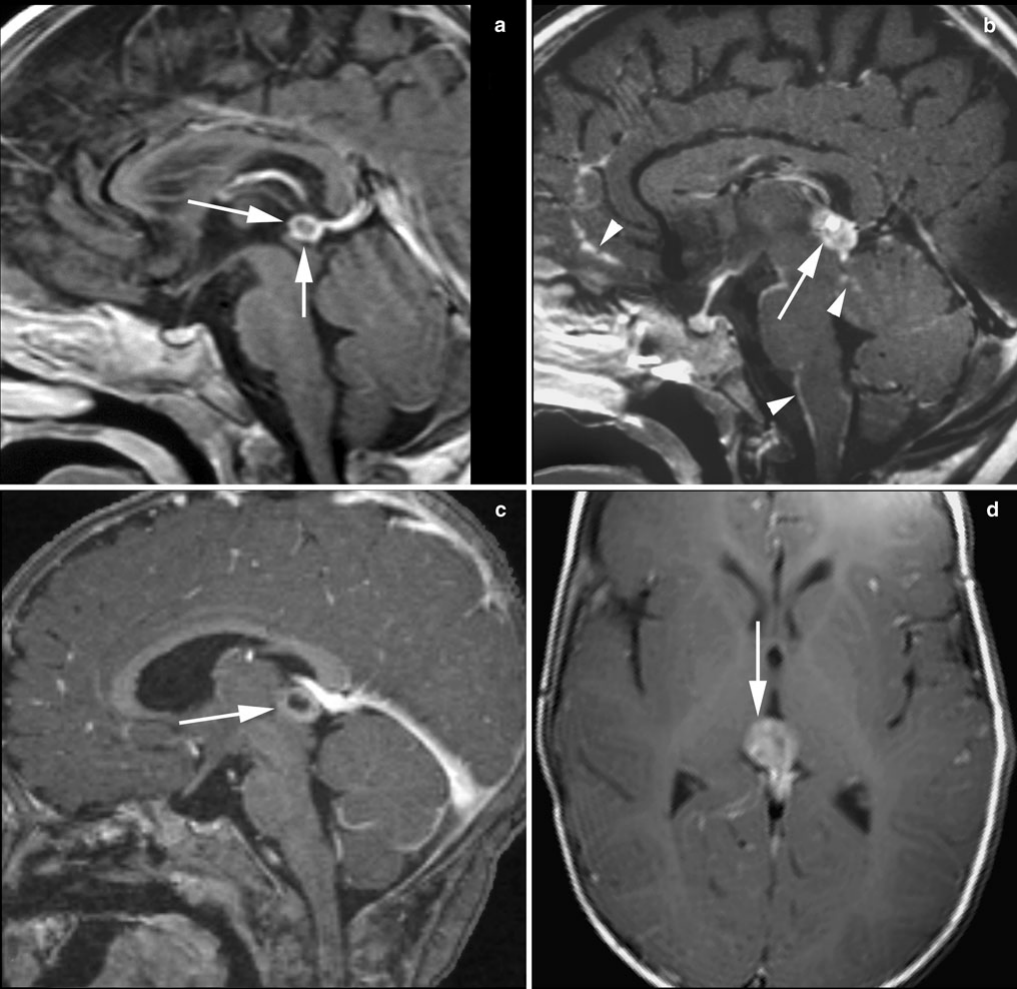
Pineoblastoma presenting as suspicious cyst. Contrast-enhanced sagittal (a–c) and axial (d) T1-weighted images of the brain in patient 6 (a, b) and patient 16 (c, d). The pineal gland in a shows an irregular cyst wall with tiny nodules, which progressed into a solid tumor with diffuse (nodular) leptomeningeal metastases 14 months later after treatment refusal (b). The pineal gland in patient 16 mimics a pineal cyst on the sagittal image (c), but shows a solid part of the lesion on the axial image (d), suspicious for pineoblastoma
